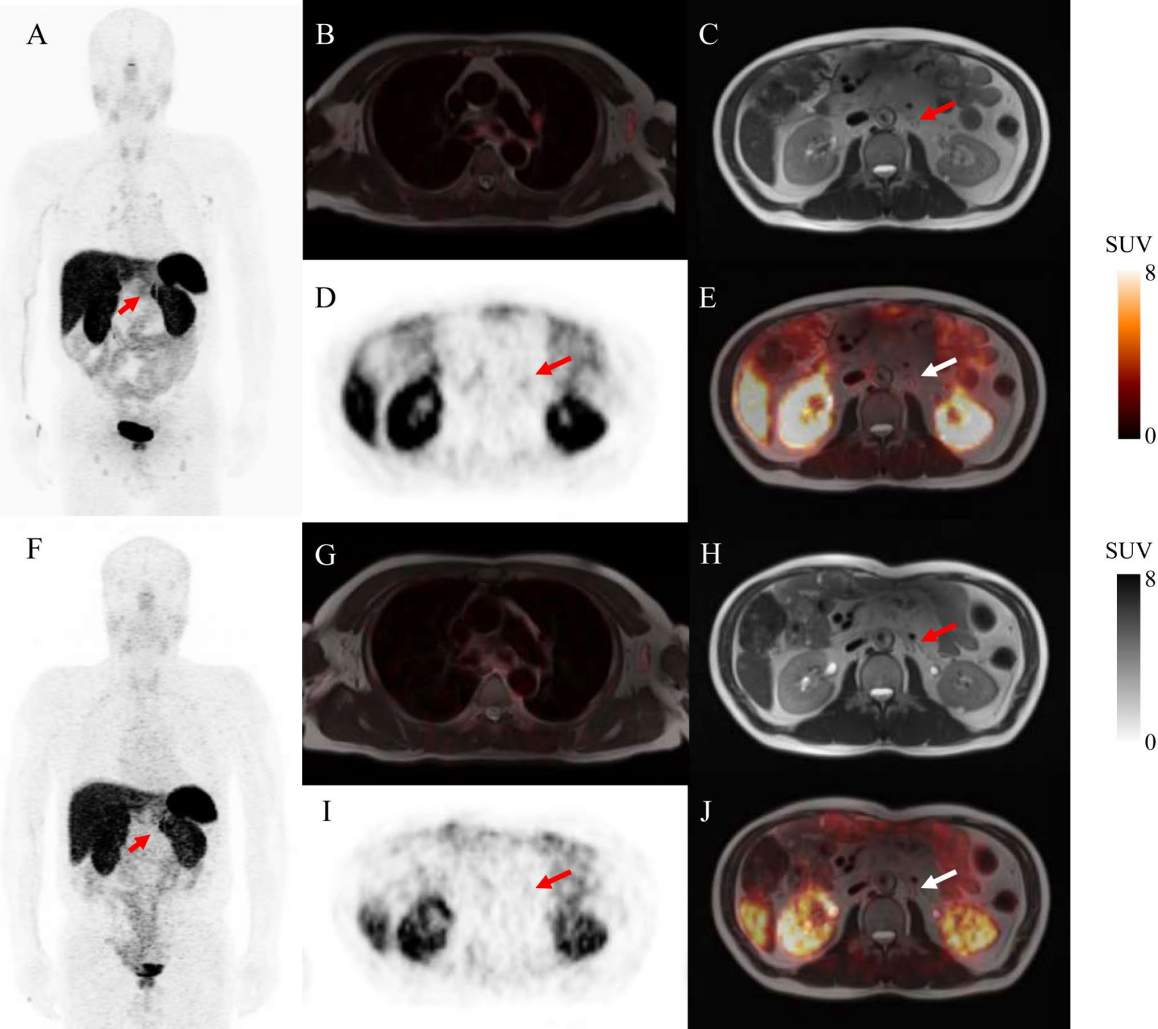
Equivocal SSTR-targeted PET/MR uptake in soft-tissue lesions. ^68^Ga-DOTATATE PET/MR imaging was performed for the restaging of a 60-year-old male with a history of pancreatic NET. Whole-body maximum intensity projection images (MIP) revealed multiple LN metastases with radiotracer uptake that was either pathologic or equivocal (A). PET/MR-fused images showing blood pool uptake with a SUVmax of 1.05 (B). Axial T2 weighted MR image (C), PET image (D), and fused PET/MR image (E) results revealed a retroperitoneal LN with only slightly higher radiotracer uptake than the blood pool (arrows, SUVmax: 2.9). Follow-up MIP (F), blood pool uptake (G), axial T2 weighted MR image (H), axial ^68^Ga-DOTATATE PET (I), and axial ^68^Ga-DOTATATE PET/MR (J) scans performed 28 months after initial imaging and treatment showed no visible radiotracer uptake on the follow-up scan (arrows), consistent with likely NET involvement
